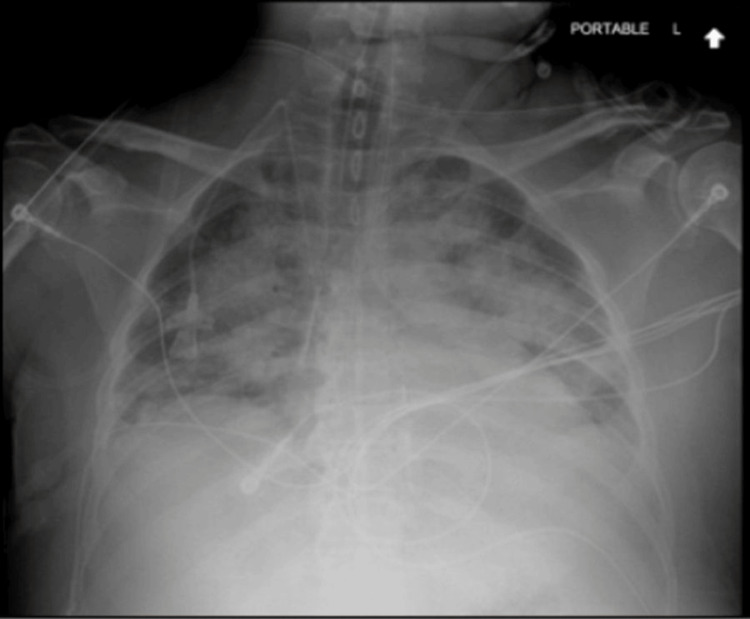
Chest X-ray showing extensive bilateral pulmonary infiltrates.
